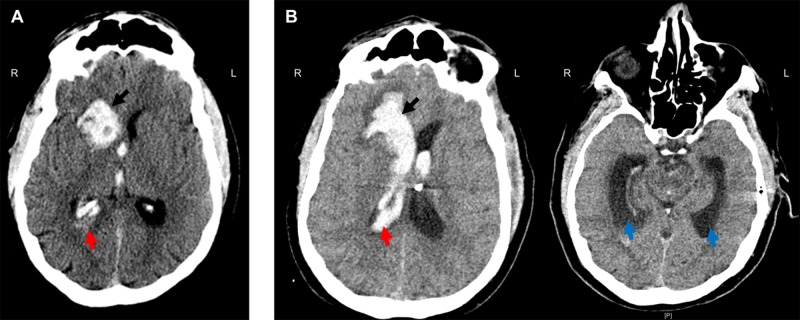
Head computed tomography (CT) pre- and post-admission. A) Right caudate intracerebral hemorrhage (ICH) (17 cm^3^) (black arrow) with ventricular extension (red arrow) but without hydrocephalus. B) Repeat CT head significant for slight increased size of ICH (21 cm^3^) (black arrow) with greater intraventricular hemorrhage (red arrow), casting of the right lateral and third ventricles, and hydrocephalus (blue arrow).
